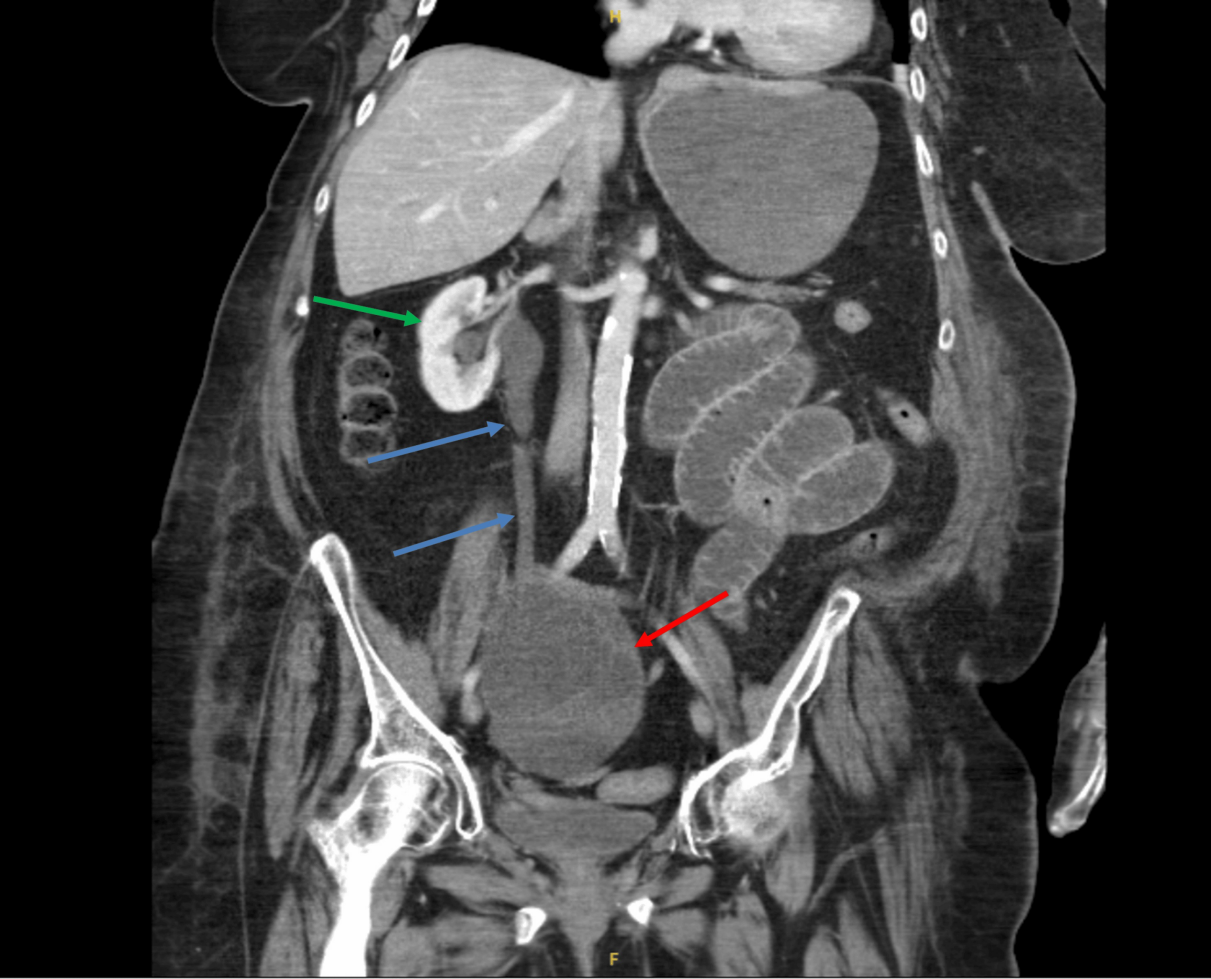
Contrast-enhanced CT scan in the coronal view reveals a cystic mass (red arrow) compressing the right ureter causing right-sided hydroureteronephrosis (bloe arrows). The right kidney shown with the green arrow.
